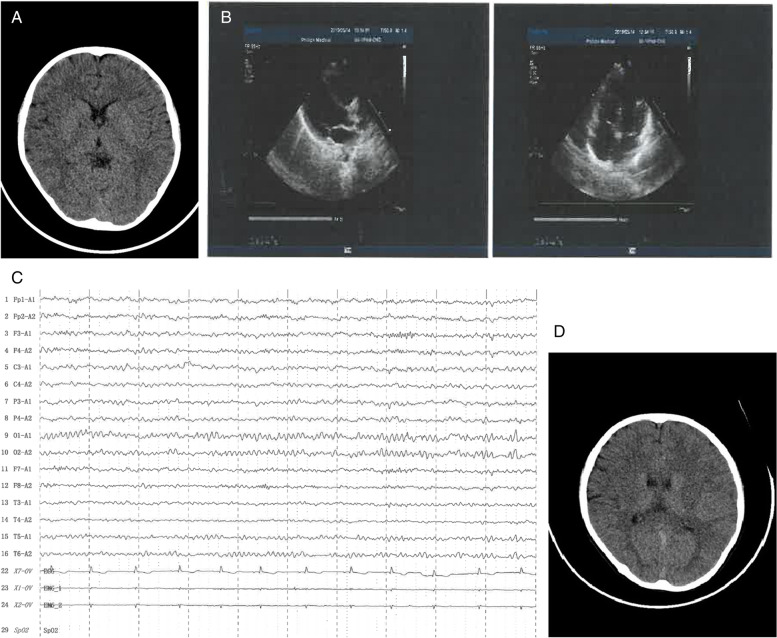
During follow-up A CT scan showed slightly high density shadow in the left occipitoparietal lobe on October 23, 2018; B Color doppler echocardiography showed that the intracardiac structure was normal, left ventricular systolic function was normal, and EF was 72% on May 14, 2019; C EEG showed no clinical events on September 26, 2020; D head CT scan showed normal image on July 9, 2021
